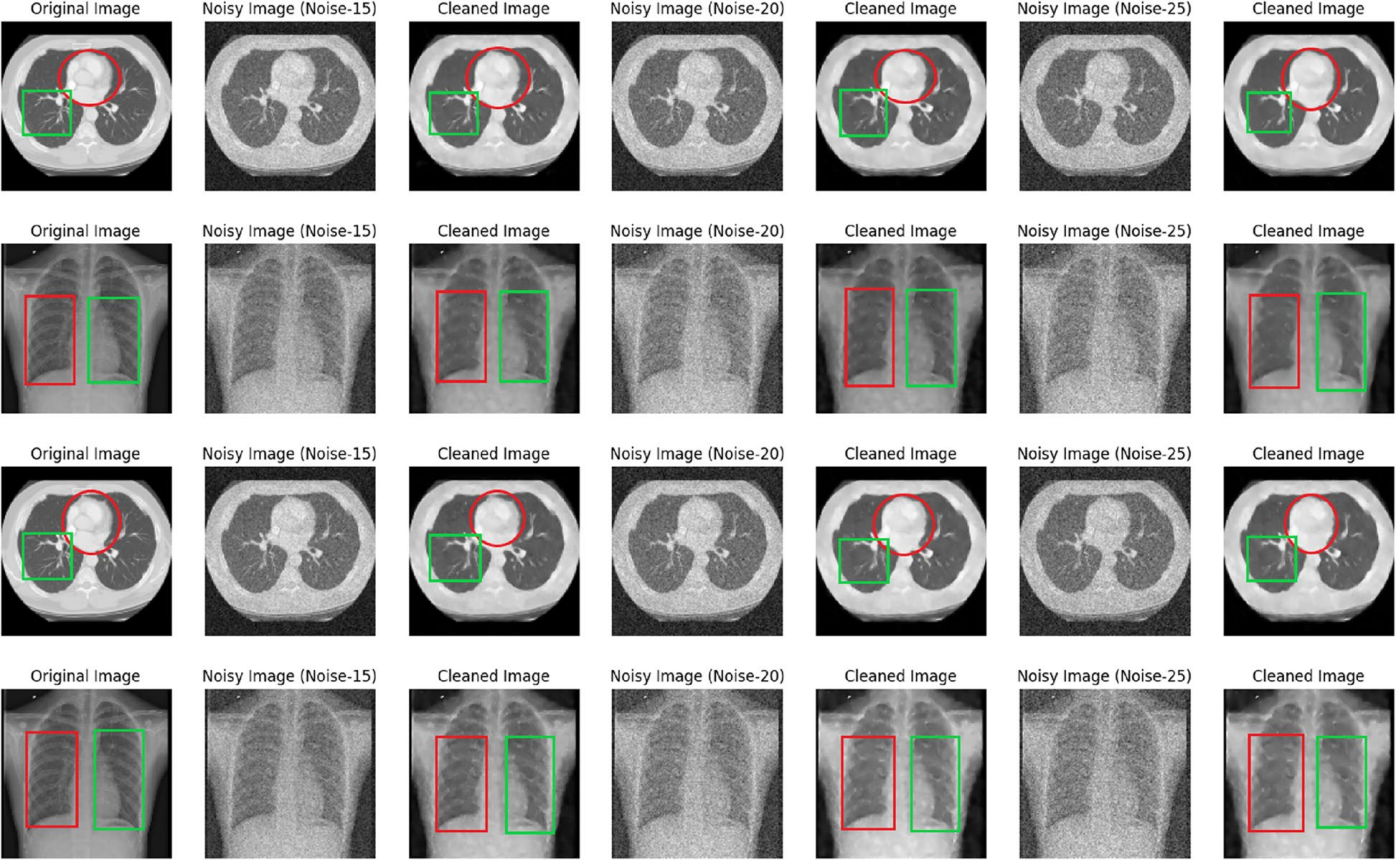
Original CT and X-Ray image with noisy($$N_{sp}$$=15,20,25 and $$N_{bl}$$=0 to 55) and denoised or cleaned image by DMF-Net where same images of Figs. 4 and 6 are shown with specific noise and blind noise
